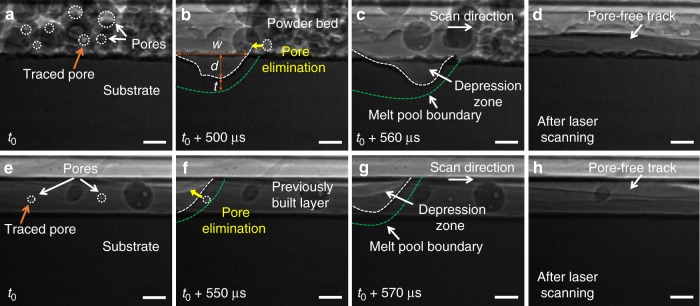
Eliminating pores using thermocapillary force. a–d Dynamic X-ray images showing elimination of pores in feedstock powders. The thickness of powder layer is 100 μm. In b
